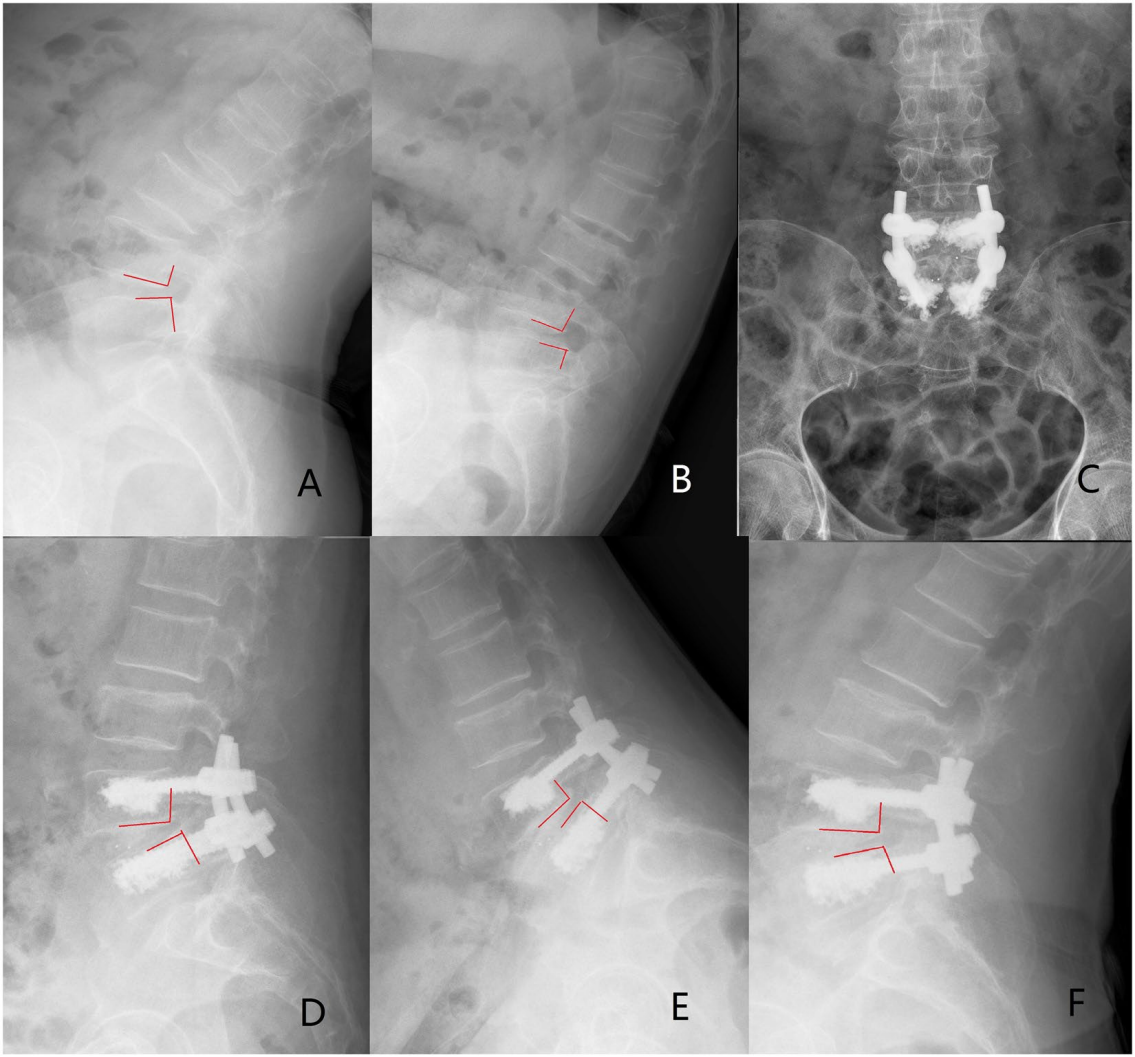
A 64-year-old female (T = −3.8) who was treated with bilateral 4 CICPSs fixation by PMMA augmentation. (A,B) Preoperative radiographs indicated the L4 spondylolisthesis. (C,D) The postoperative radiographs showed lumbar sequence reconstruction. (E,F) CICPSs was observed in place after 6 months of surgery and bony fusion was achieved.
